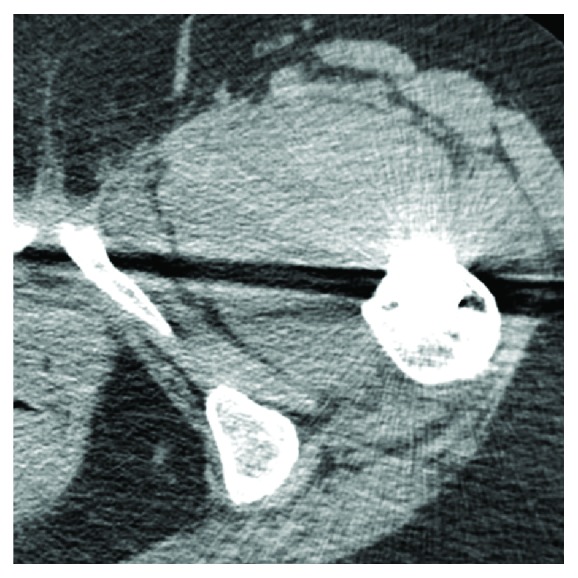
Axial section of CT scan showing hematoma in iliopsoas region.
